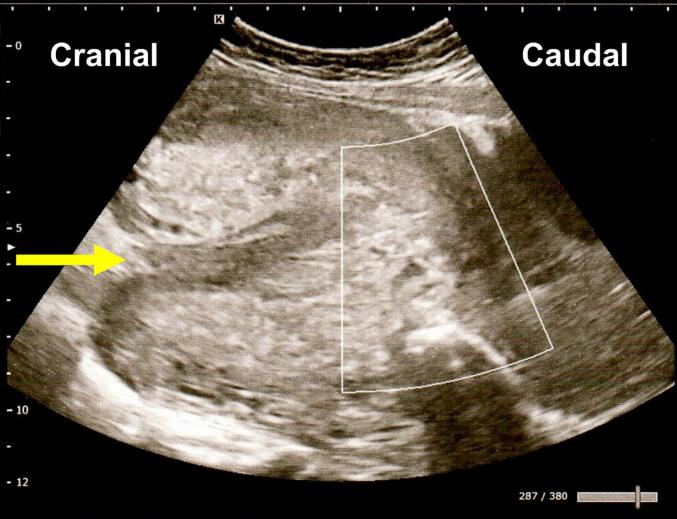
Sagittal transabdominal ultrasound image during uterine inversion. The fundal depression caused by the inversion (arrow).
